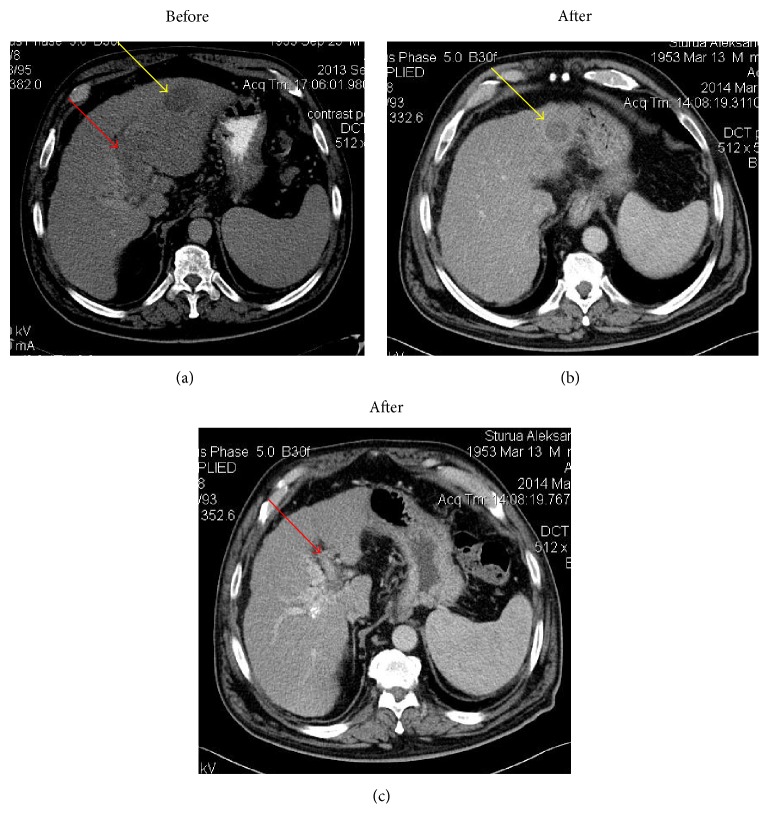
Comparison: CT before and in 5 months after VesOpen procedure. LPV (red arrow) is recanalized. The residual tumor is represented only by hypodense component, the size of which is decreased (yellow arrow).
